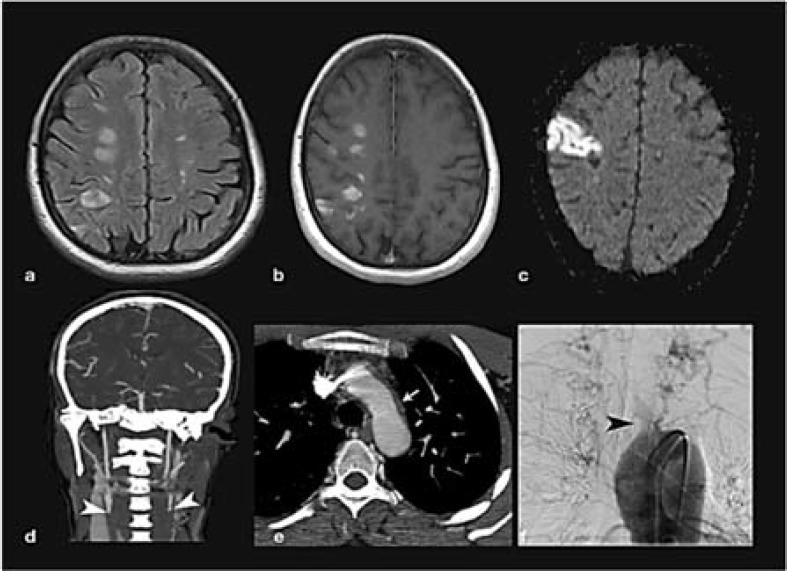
Imaging findings of the patient; magnetic resonance imaging (MRI) at the first attack, axial T2 fluid attenuated inversion recovery (a), and T1 post-contrast (b) sequences showing multiple foci of gadolinium enhancing and non-enhancing signal changes in the periventricular and subcortical white matter. Diffusion weighted MRI at the second attack (c) revealed an area of restricted diffusion in right parietal cortex indicative of an ischemic lesion. Coronal view computed tomography (CT) angiography of brain and neck at the second attack (d) showing occlusion of bilateral common carotid arteries (white arrowheads). Axial CT angiography at the level of aortic arc at the second attack (e) showing thickening of the aortic wall (arrow). Digital subtraction angiogram at the second attack (f) revealing complete occlusion of the main branches of the aortic arc (dark arrowhead).
